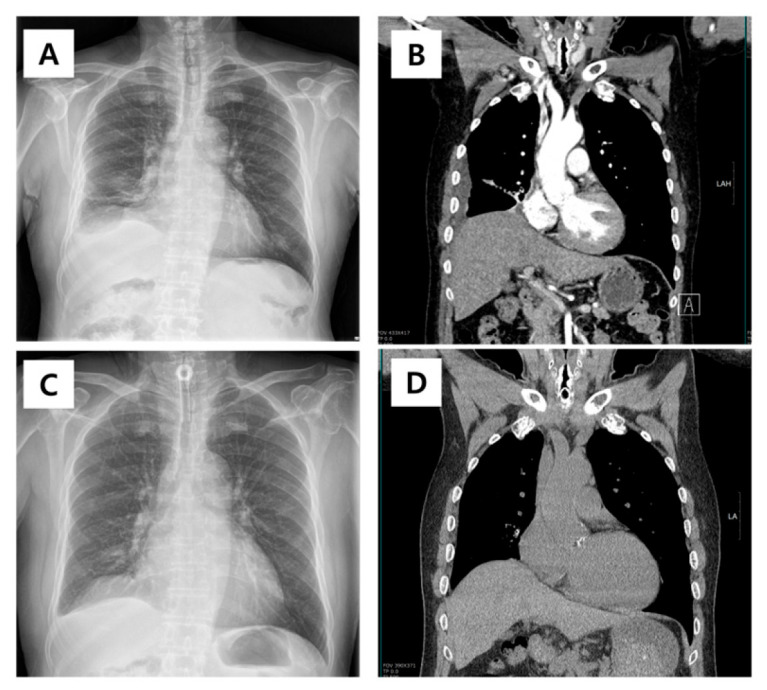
Radiographic and computed tomography (CT) findings before and after tracheostomy in a patient with tracheobronchopathia osteochondroplastica. (A) Initial chest radiograph showing partial atelectasis in right lower lobe; (B) initial chest CT scan demonstrating airway narrowing and tracheobronchial calcification, predominantly involving the upper trachea and extending to the level of the vocal cords; (C) chest radiograph after emergency tracheostomy, with tracheostomy tube in situ; (D) follow-up CT scan after tracheostomy.
